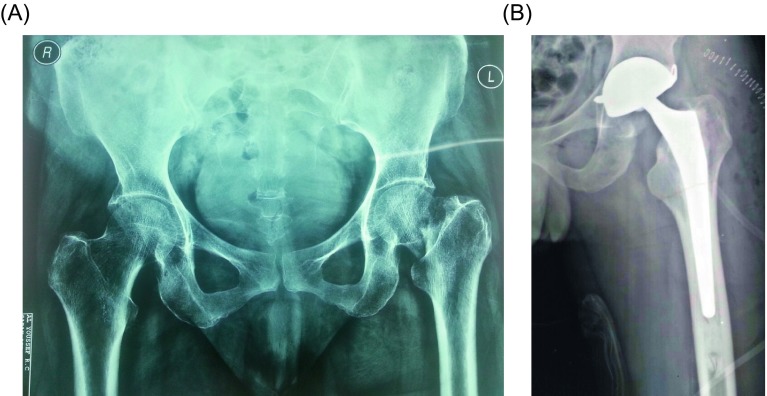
(A) Preoperative radiography of a 65 years old female patient with a fracture of the left femoral neck, (B) postoperative X-ray of a cemented dual mobility THA, the superior fixation screw was left due to the press fit metal cup.
